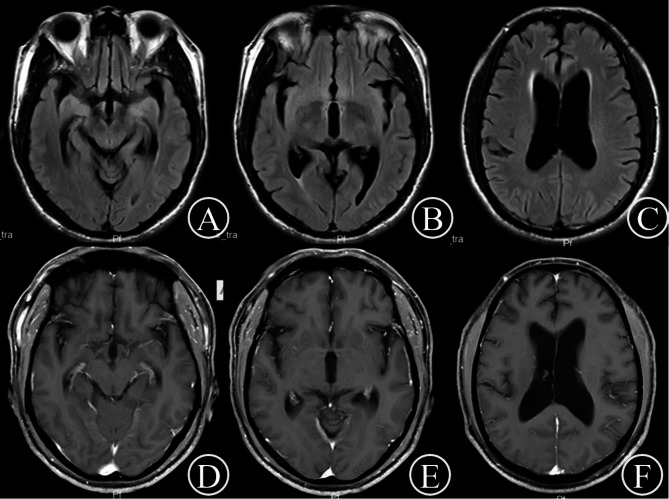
Brain MRI revealed mild brain atrophy in the frontal and temporal lobes and hippocampi and mild supratentorial hydrocephalus (A, B, C); no enhancement was observed after gadolinium injection (D, E, F)
